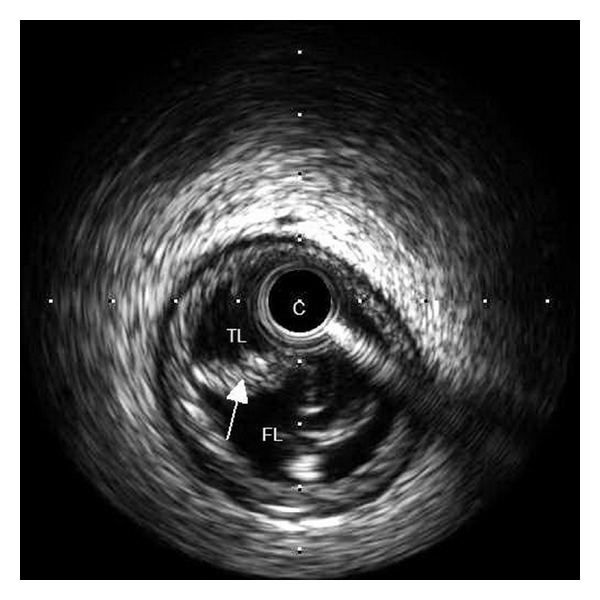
IVUS of the RCA showing evidence of coronary artery dissection (intimal flap marked with white arrow) which delineates the true and false lumens. C: IVUS catheter; FL: false lumen; IVUS: intravascular ultrasound; RCA: right coronary artery; TL: true lumen.
